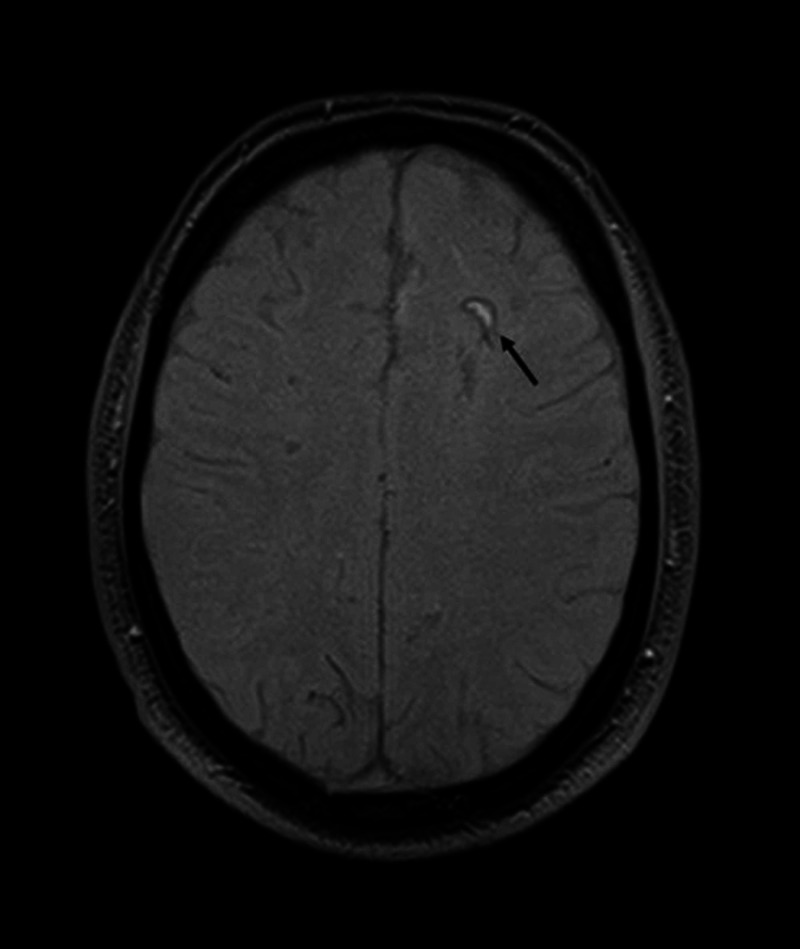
Hemorrhagic transformation of acute infarct on initial brain MRI The black arrow demonstrates a crescent-shaped hyper-intense area with peripheral susceptibility artifact in the left frontal lobe on the axial GRE sequence. Findings are considered consistent with the hemorrhagic transformation of the acute ischemic infarct. GRE - gradient echo
